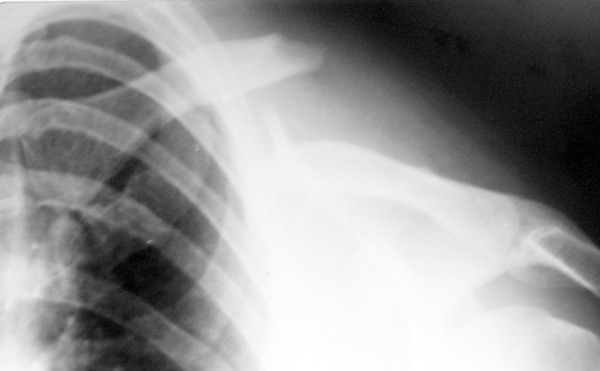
Radiography showing fracture of the clavicle.
